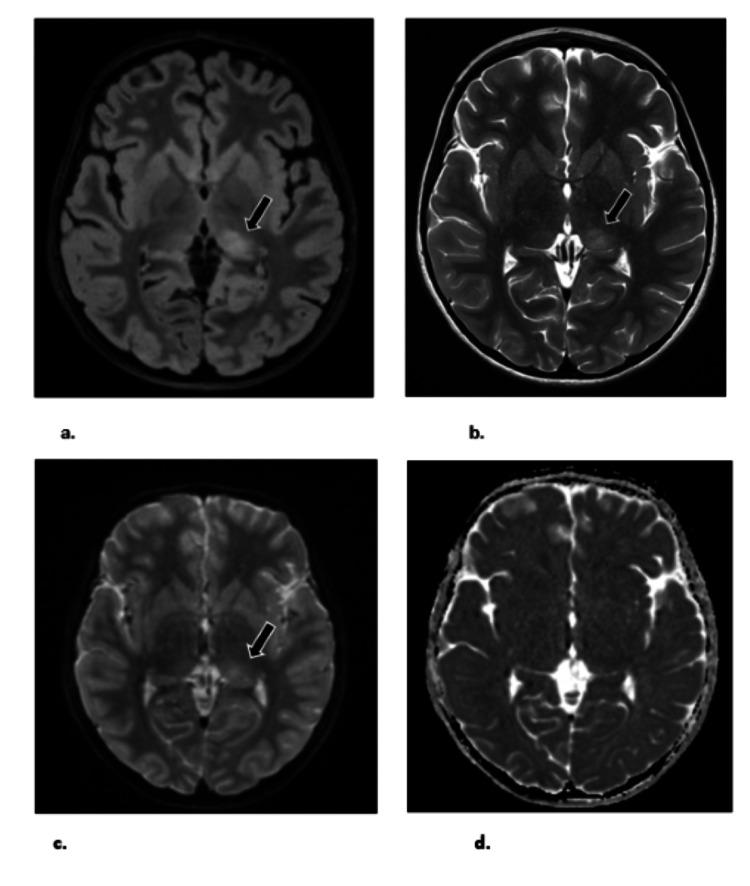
Brain MRI showed T2/FLAIR hyperintensity in left thalamus a. FLAIR, b. T2, c. DWI, d. ADC. FLAIR = fluid-attenuated inversion recovery; T2 = T2 weighted image MRI; DWI = diffusion-weighted imaging; ADC = apparent diffusion coefficient.
